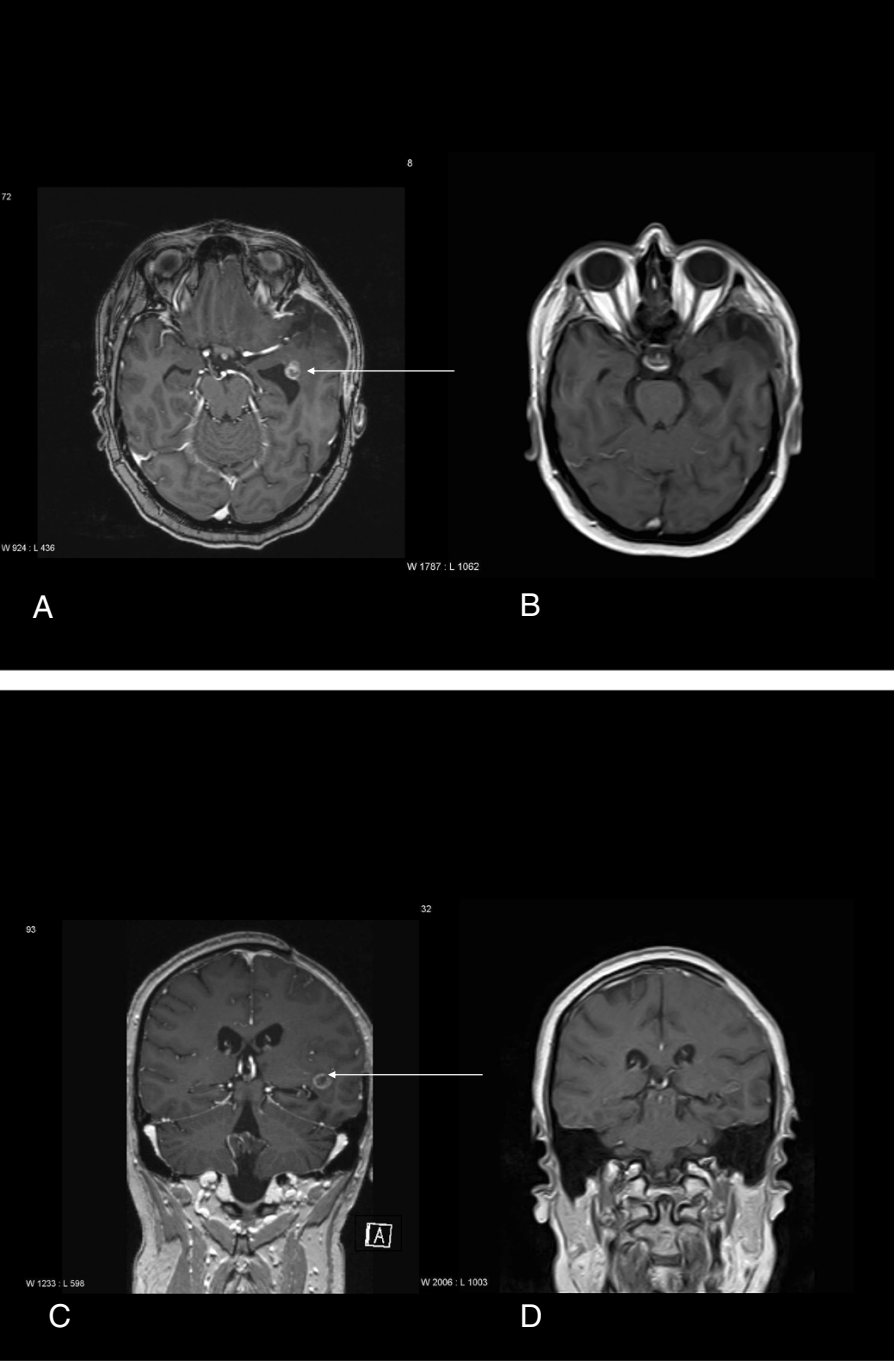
MRI slides of patient with major response. T1 weighted, contrast enhanced MRT. A: Horizontal: before start of imatinib: with a left frontal lesion with contrast enhancement. B: Horizontal: 3 months after start of imatinib, contrast enhancement of the lesion is not longer visible. C: coronal, before start of imatinib with the contrast enhancing lesion near the ventricle. D: coronal, 3 months after start of imatinib: no contrast enhancing lesion visible. The best fitted sections were selected for this image, as the head positioning and bending of the neck were not exactly similar in both examinations.
